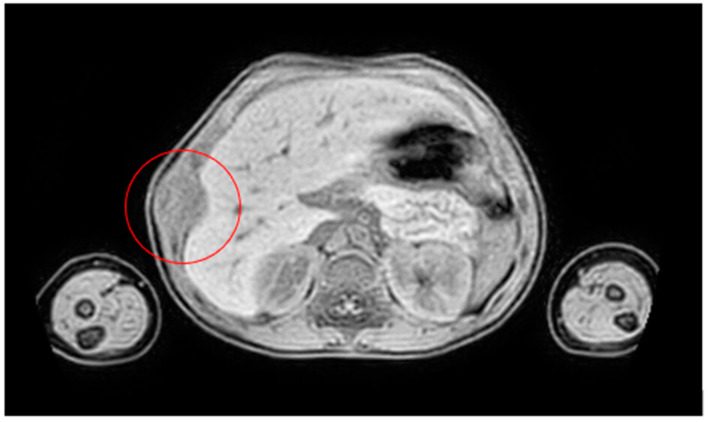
MRI at the presentation.
